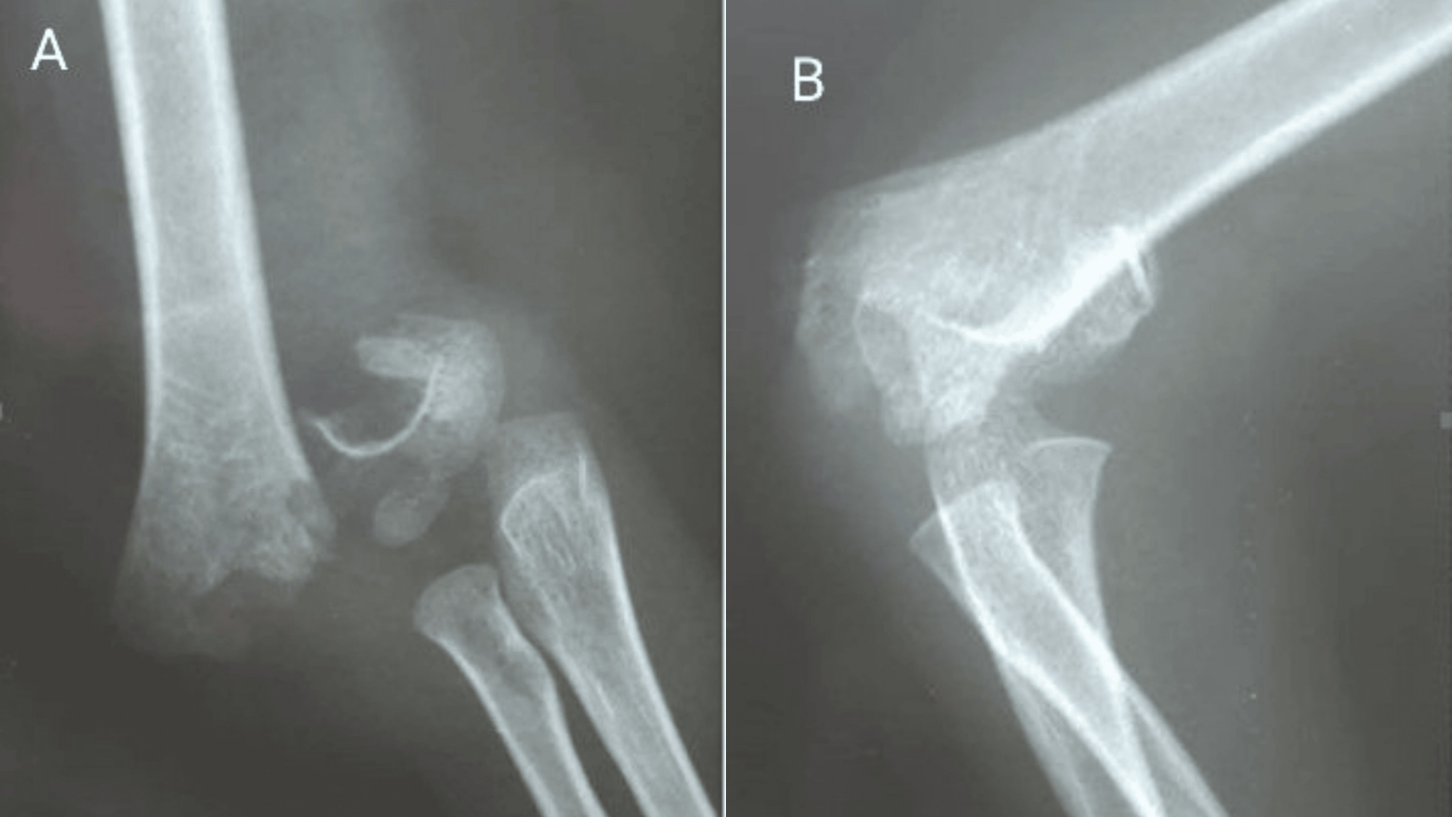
Right elbow X-rays, showing a type III supracondylar fracture A: Anteroposterior view. B: Lateral view
